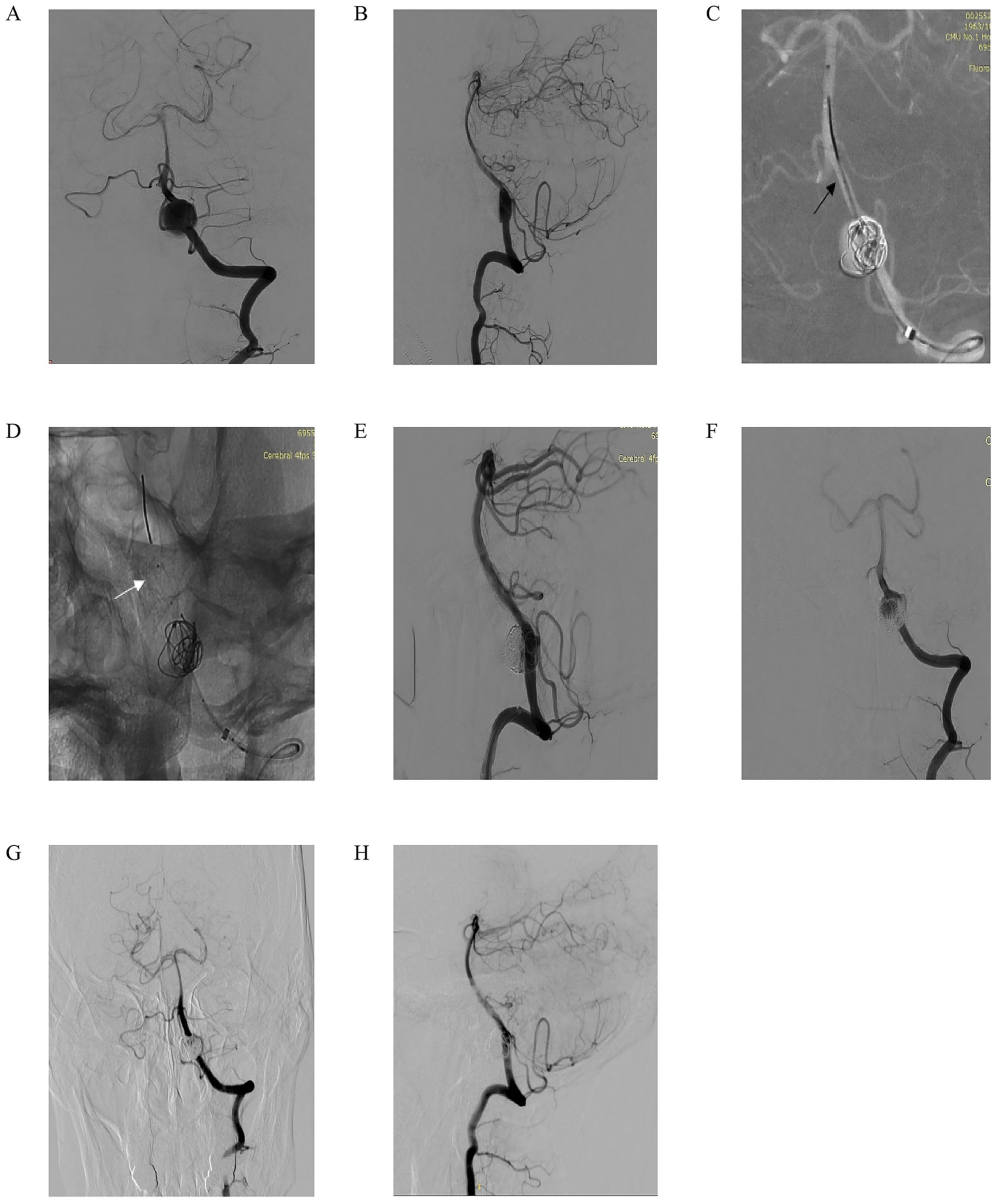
A 60-year-old man intermittently experienced headaches for 2 months. There was no nausea or vomiting, and no choking while drinking water. Computed tomography angiography (CTA) indicated an aneurysm in the left V4 segment. No positive signs were found in the specialized physical examination. (A,B) Left vertebral artery DSA in anteroposterior (A) and lateral (B) views confirming the presence of a fusiform aneurysm at the V4 segment; (C) Working-angle roadmap guidance for navigation of a 6F intermediate catheter and microcatheter (XT-27) to the target site. The black arrow marks the distal deployment landmark of the Lattice FD-MB; (D) Partial coiling of the aneurysm sac with the Lattice FD-MB maintained in a semi-deployed state. The white arrow highlights the optimal distal expansion of the device; (E,F) Post-coiling DSA in anteroposterior (E) and lateral (F) views demonstrating substantial aneurysm filling with coils, stable expansion of the Lattice FD-MB, preserved hemodynamics, and unimpaired flow into the left posterior inferior cerebellar artery (PICA); (G,H) Seven-month follow-up DSA in anteroposterior (G) and lateral (H) views confirming complete aneurysm occlusion, patency of the parent vessel without stenosis, and maintained perfusion of the PICA.
